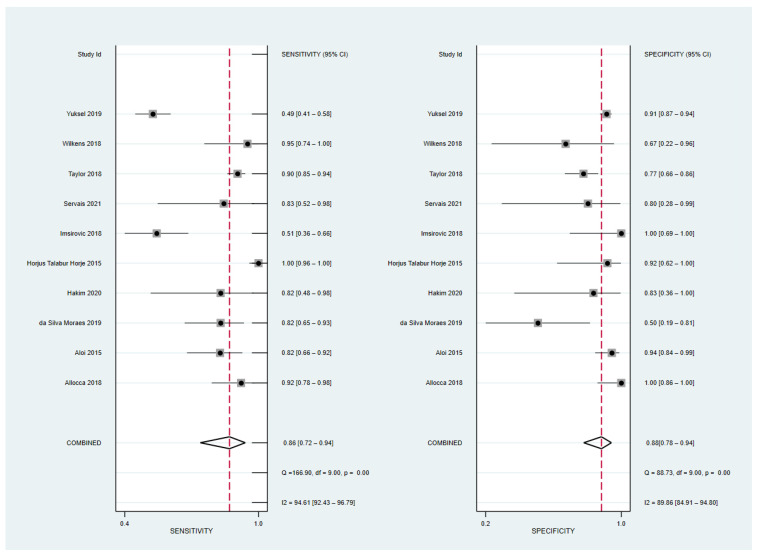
Coupled forest plots of overall pooled sensitivity and specificity for diagnosing active bowel lesions in patients with Crohn’s disease using ultrasonography [17,18,19,20,21,22,24,25,26,27].
